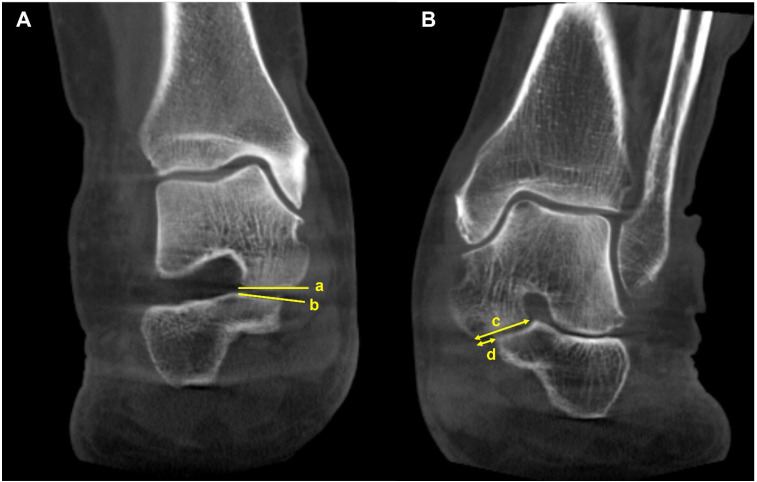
Middle facet subluxation. (A) Measurement of the incongruence angle of the middle facet of the subtalar joint on a coronal-plane weight-bearing CT image, which is an angle between both articular surfaces (a and b). (B) Measurement of the percentage of “uncoverage” of the middle facet of the subtalar joint on a coronal-plane weight-bearing CT image. (c) = the width of the talar middle facet, and (d) = the linear measurement of the middle facet uncoverage. The percentage of uncoverage of the middle facet of the subtalar joint = (d/c).
